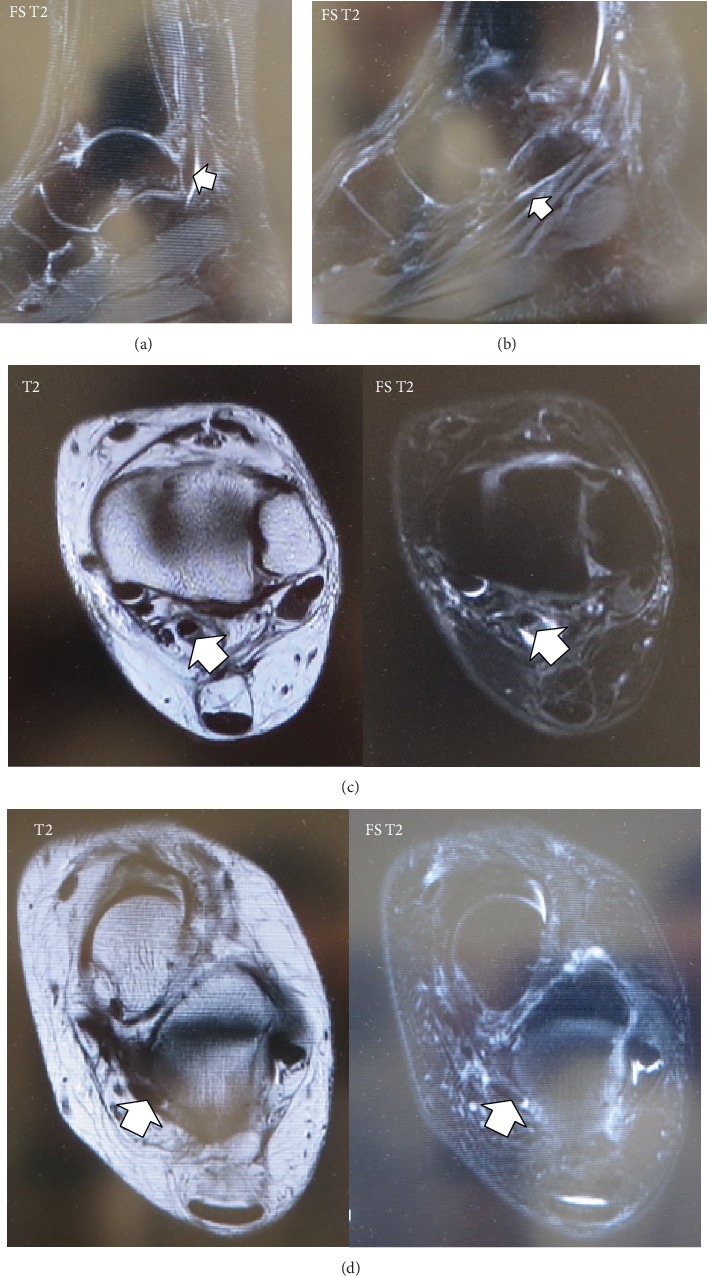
Fat saturation T2-weighted magnetic resonance images showing no effusion along the flexor hallucis longus (FHL) tendon at a level proximal to the ankle joint posteriorly in the (a) sagittal and (b) axial planes. T2-weighted and fat saturation T2-weighted images showing no accumulation of effusion along the FHL between the talar tubercles and the master knot of Henry in the (c) sagittal and (d) axial planes.
